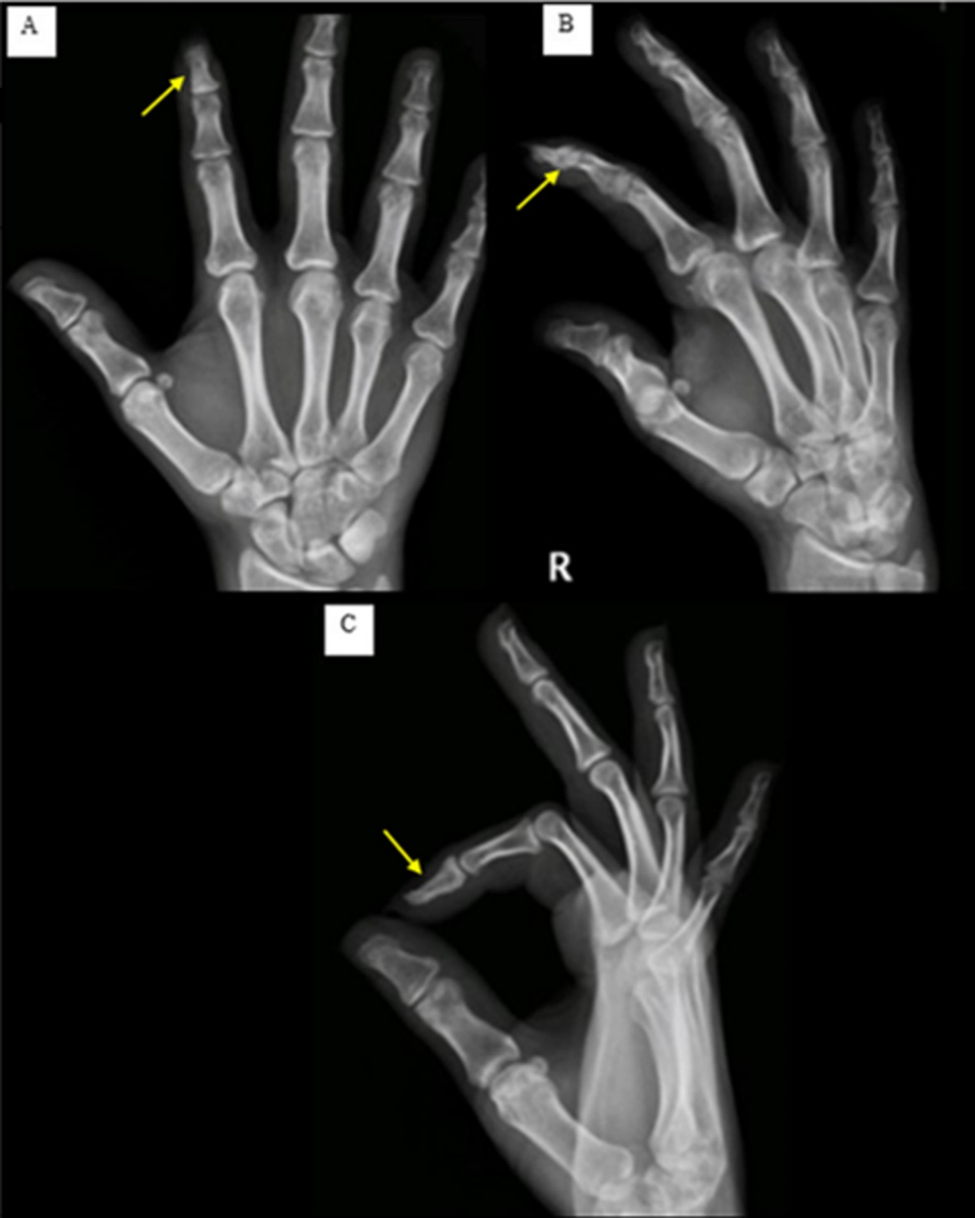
Follow-up radiographs at nine months post-operative showing a decrease in size of the cyst involving the distal phalanx of the right index finger with a relative increased sclerosis on (A) AP, (B) oblique, and (C) lateral views (yellow arrows). AP, anteroposterior.
